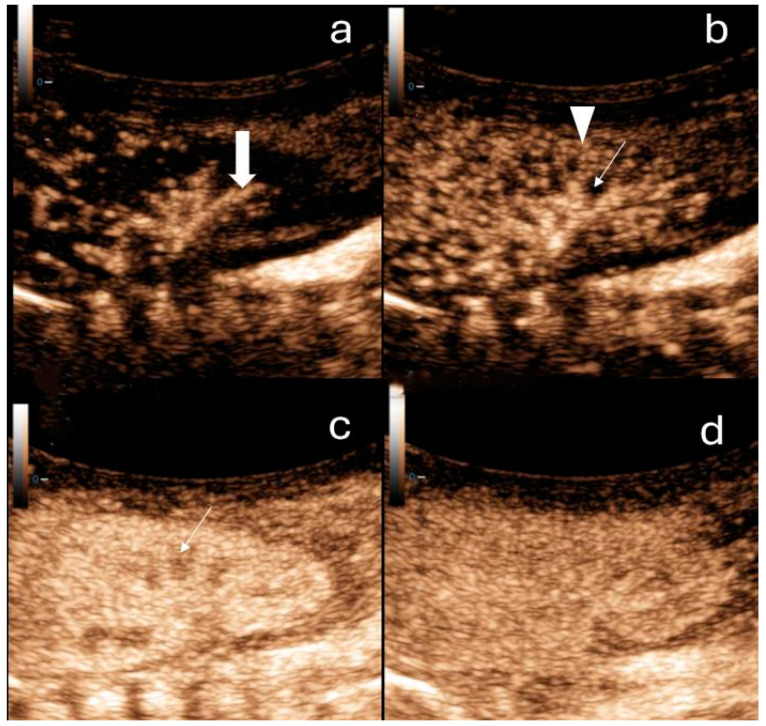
Contrast-enhanced ultrasound of the right kidney in a newborn. (a) Arterial phase: a few seconds after contrast administration interlobar arteries of the kidney can be observed (thick arrow). (b) Cortical phase: a few seconds after the arterial phase the contrast distributes within the renal cortex (arrowheads). The medulla (arrow) is not enhancing. (c) Parenchymal phase: after approximately 20 s, the outer medulla is filled with contrast (arrow). (d) In the late phase (2 min after contrast application), the pyramids are fully enhanced.
